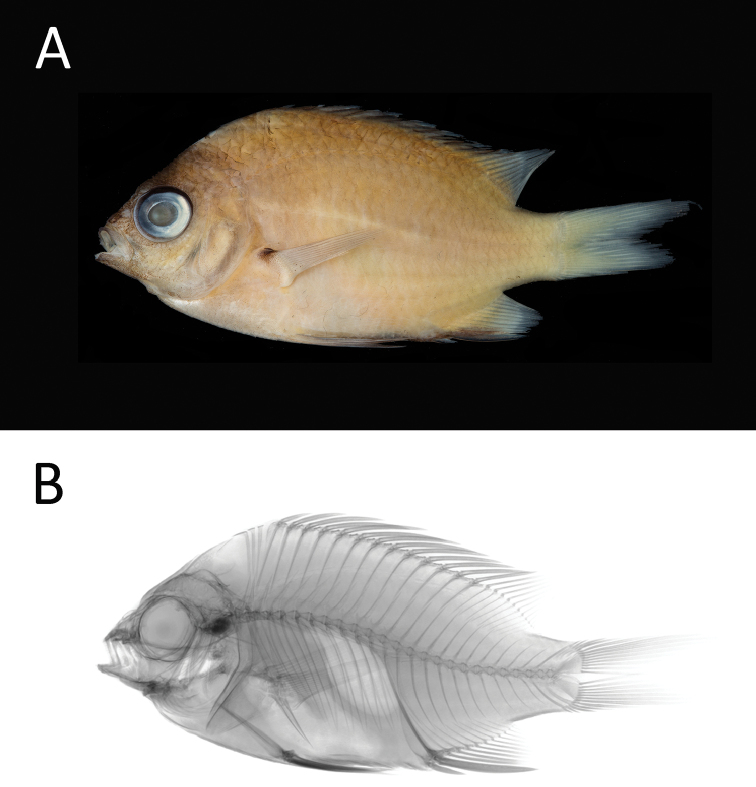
Chromishangganan* sp. n. PNM 15358 a holotype preserved in alcohol, 57.75 mm SLb radiograph (photographs JD Fong).
